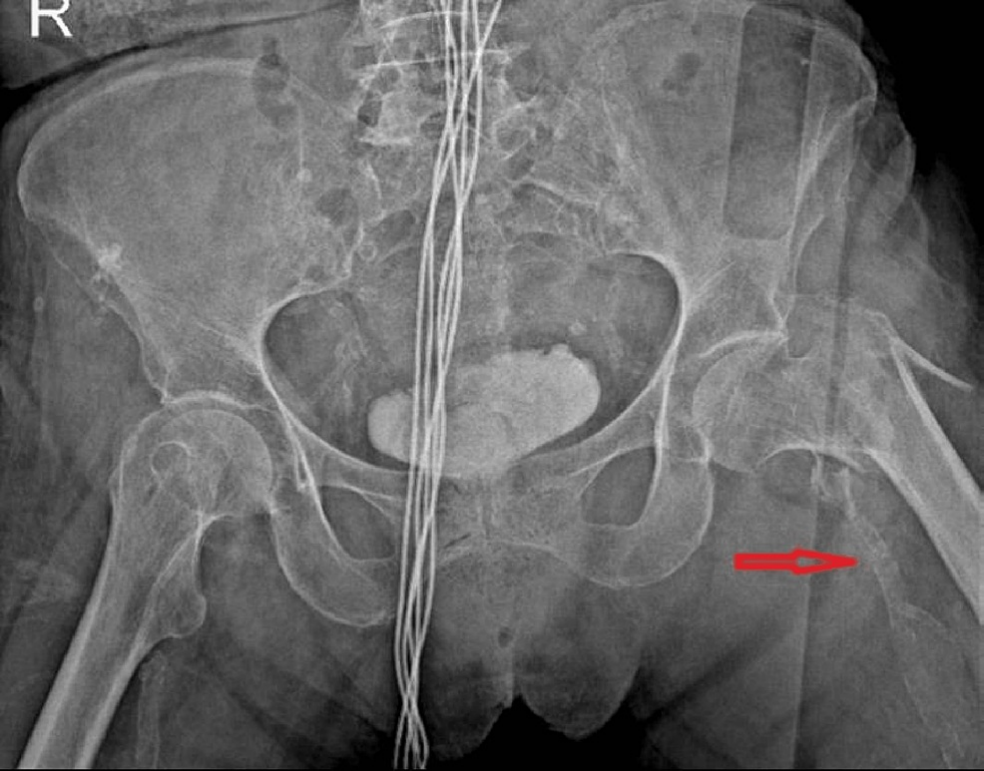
Radiological image of the femoral artery calcification on the fractured side in pelvis anteroposterior (AP) X-ray
